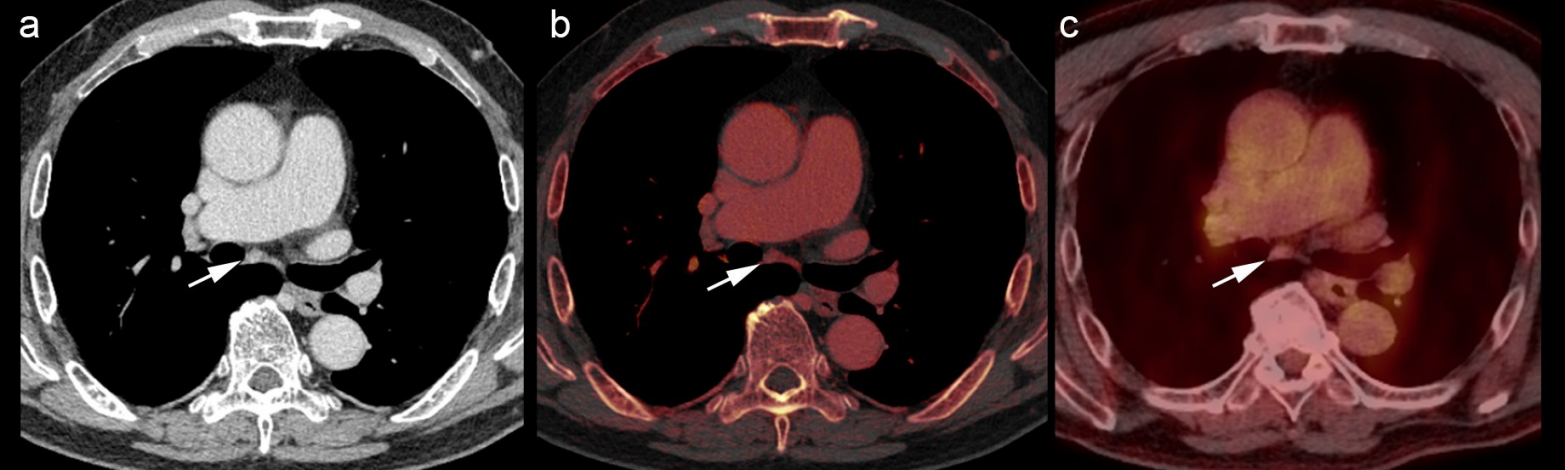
Representative dual-energy CT and PET images in patients without lymph node metastasis. Contrast-enhanced CT image (a), iodine map (b), and PET image (c) of a 76-year-old man with esophageal cancer; images show the presence of subcarinal lymph node (a, b, c arrows), which was demonstrated as nonmetastatic postoperatively. The high iodine concentration (3.35 mg/mL) and low maximal standardized uptake value (1.4) were measured. CT: computed tomography; PET: positron-emission tomography.
